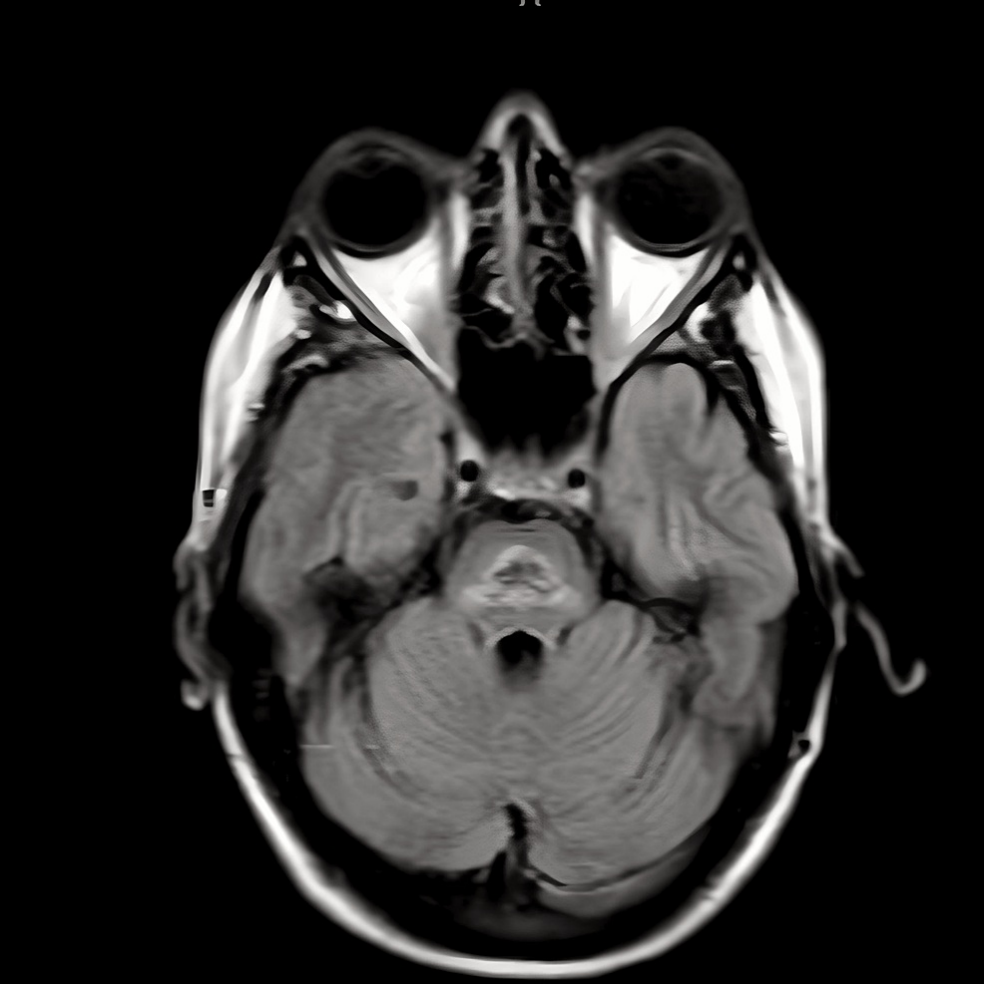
Magnetic resonance imaging of the brain
